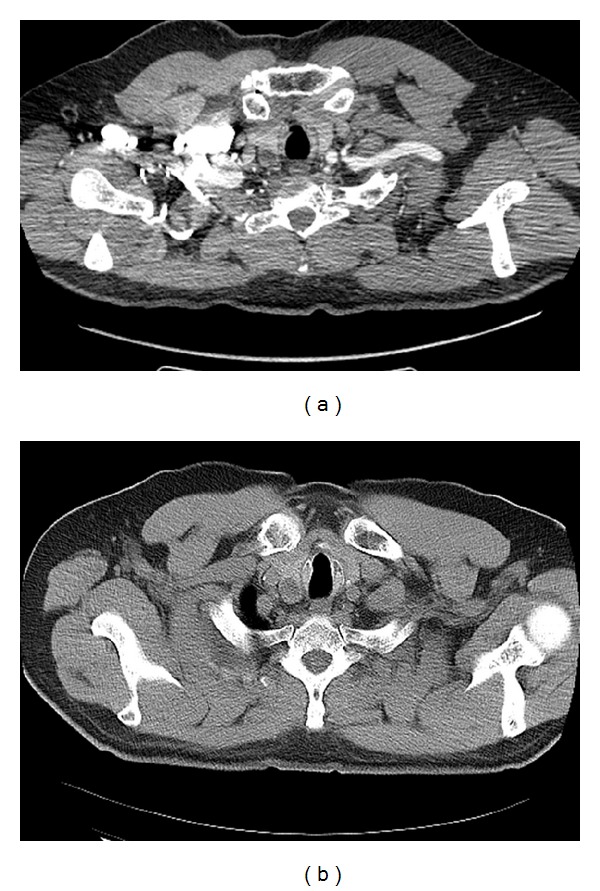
CT chest: thyroid nodule in the neck: (a) on 26 March, 2011; (b) on 24 July, 2012.
